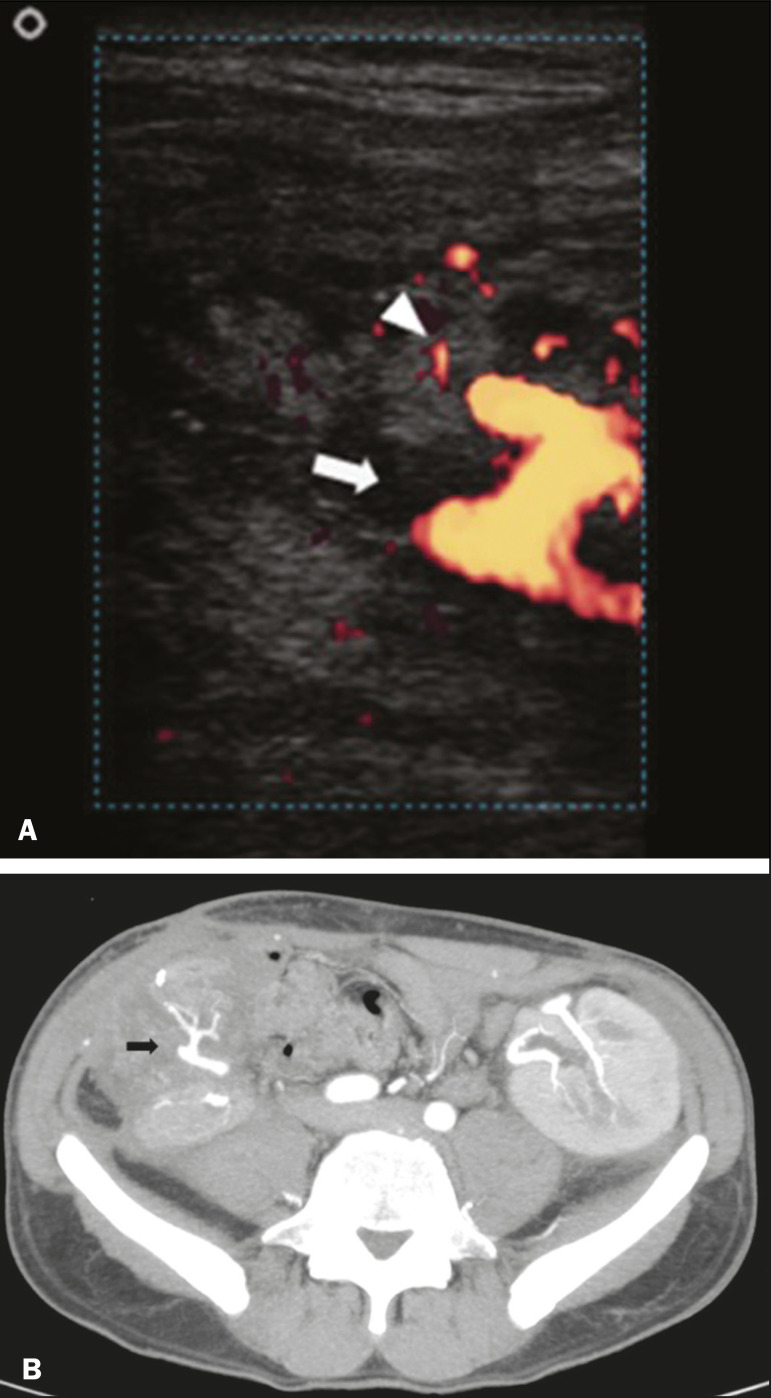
A: Cross-sectional image obtained by ultrasound in power Doppler mode, showing abrupt interruption of flow in the distal segment of the superior mesenteric artery (arrow) of the pancreatic graft, with adjacent collateral circulation (arrowhead), a finding consistent with arterial thrombosis. B: Correlation with contrast-enhanced CT in the same patient, with maximum intensity projection in the arterial phase, identifying the same abrupt interruption and a filling defect in the distal segment of the superior mesenteric artery of the pancreatic graft (arrow), confirming the arterial thrombosis suggested by the ultrasound finding.
